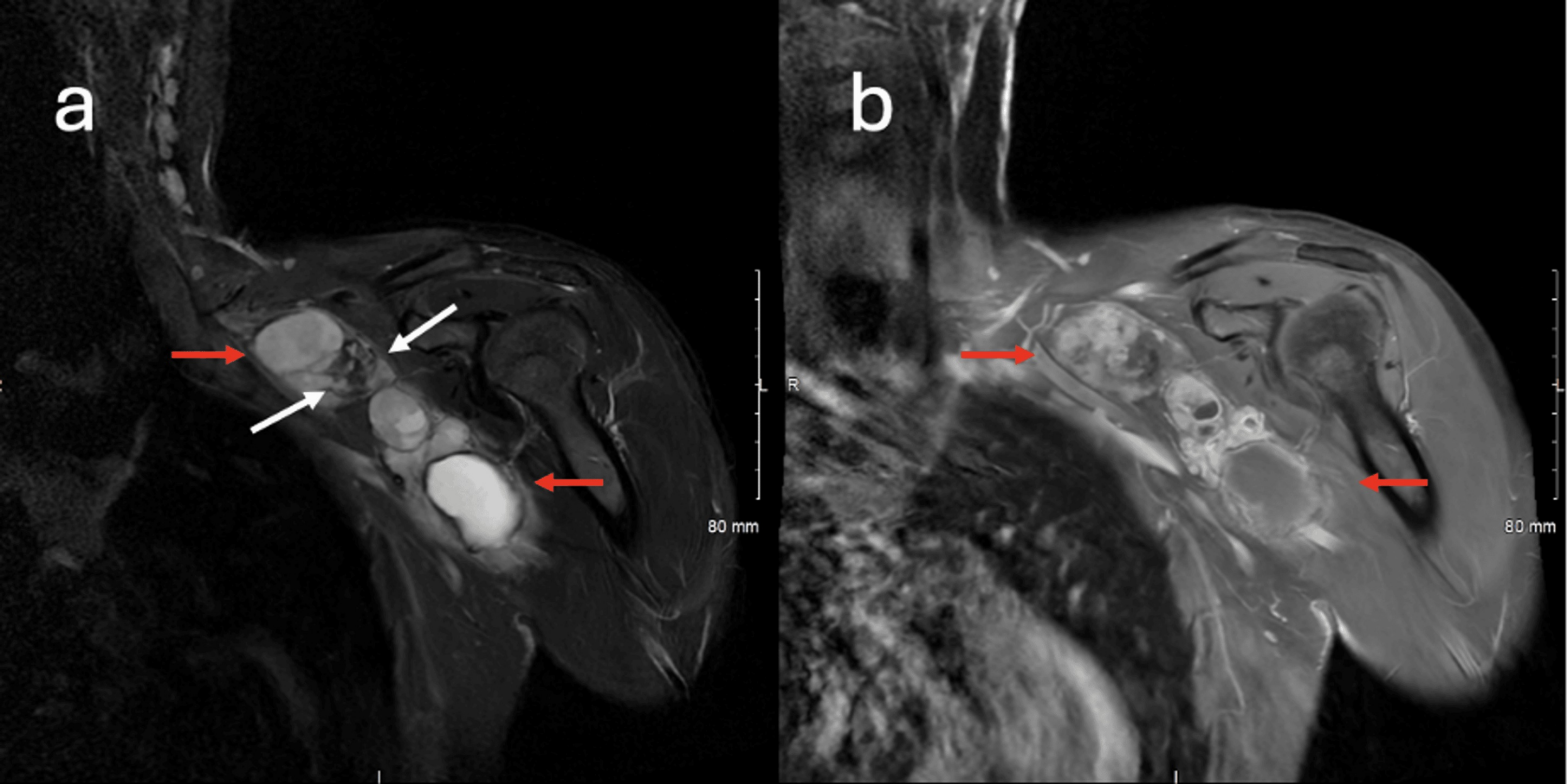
Coronal T2 STIR and post-contrast T1 fat-saturated MRI of the left brachial plexus demonstrating a heterogeneous lesion and variable cyst enhancement Coronal T2 STIR image of the left brachial plexus (a) demonstrates the complex heterogeneous lesion (red arrows) with blood products (white arrows), presumed secondary to hemosiderin deposition. Coronal T1 fat-saturated post-contrast image at the same level (b) demonstrates areas of enhancement within the lesion. Note that the more cephalad cyst (upper red arrow) demonstrates enhancement, while another area of cystic change (lower red arrow) demonstrates minimal enhancement.
